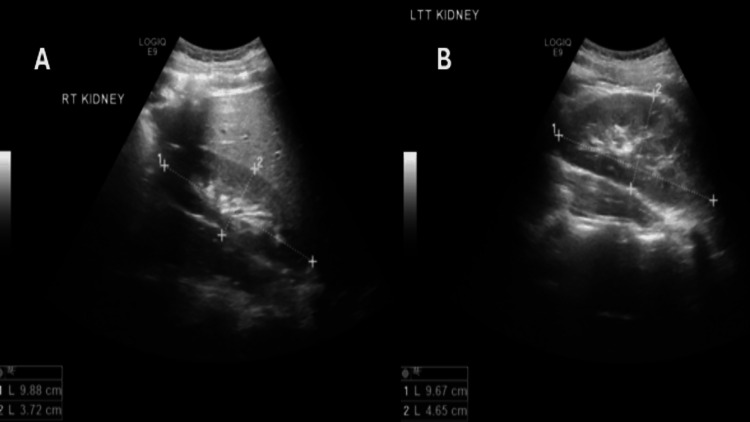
Renal ultrasound. (A) Right kidney; (B) left kidney: showing bilaterally normal-sized kidneys with mildly increased cortical echogenicity, preserved corticomedullary differentiation, and no hydronephrosis, supporting a non-obstructive, potentially reversible cause of acute kidney injury.
